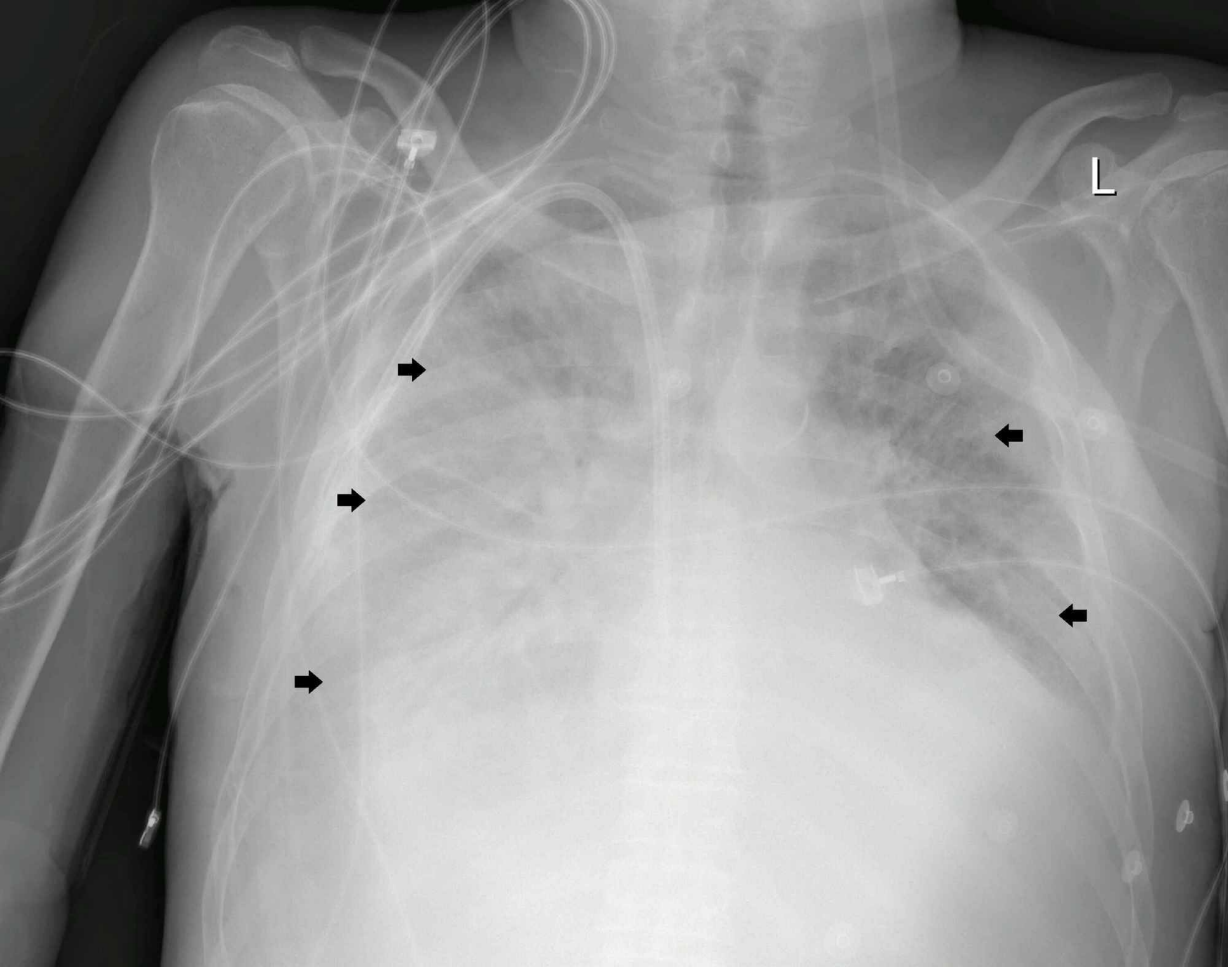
portable chest x-ray (24 hours after admission) showing bilateral patchy lower lobe predominant airspace opacification (Black Arrows).
